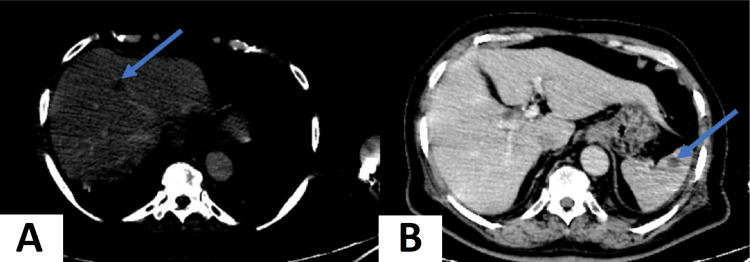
The thoracic-abdominal-pelvic computed tomography (CT) scan reveals well-defined rounded splenic and hepatic nodules without enhancement. (A) Axial section in the injected abdominal CT scan displays a rounded, well-limited, and not enhanced hepatic nodule in segment IV (arrow). (B) Axial slice in the injected abdominal CT scan depicts well-defined, rounded splenic nodules without enhancement (arrow).
